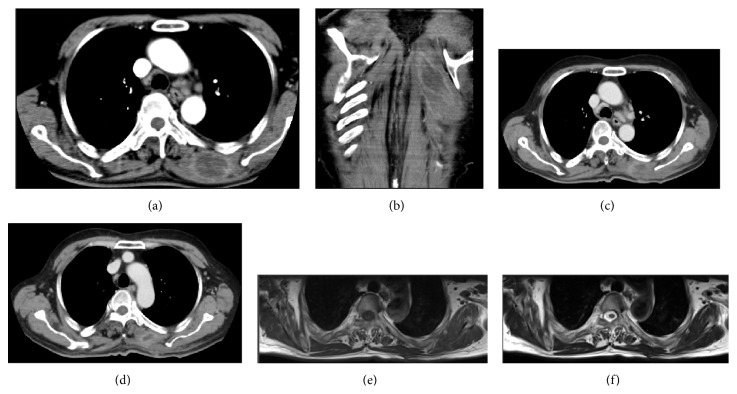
CT and MRI images. (a) Transverse section images of CT on admission showing the swelling of the left rhomboideus muscle and the presence of solitary LDA around a contrast enhancement in this muscle. (b) Coronal section images of CT on admission showing the presence of solitary LDA around a contrast enhancement in the left rhomboideus muscle. (c, d) Transverse section images of CT after drainage showing the residual LDA in the left rhomboideus muscle. (e) T1 weighted MRI images showing no abnormalities in the left rhomboideus muscle. (f) T2 weighted MRI images showing no abnormalities in the left rhomboideus muscle.
